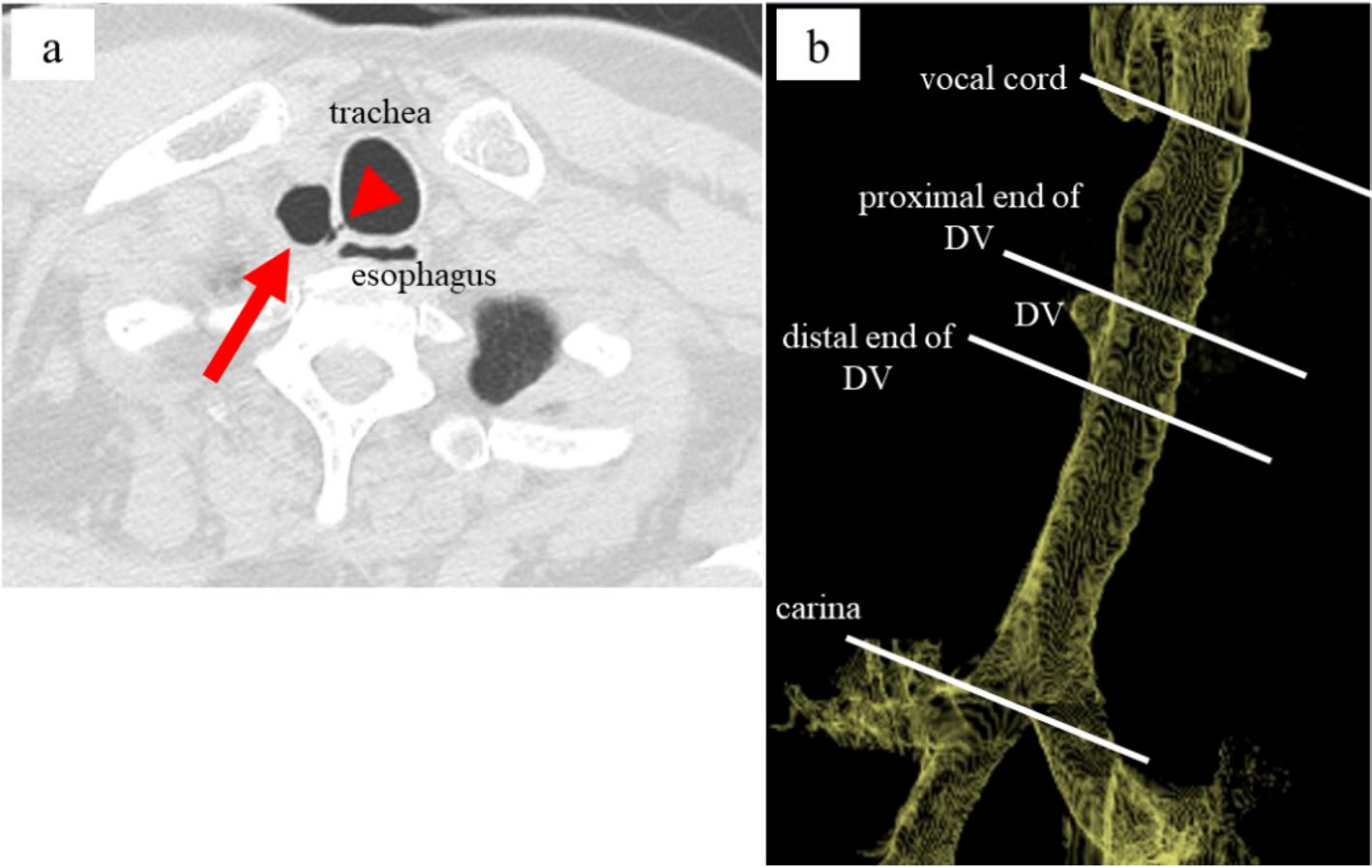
An example of CT image of the tracheal diverticulum and trachea in this study. a Axial view of chest CT image of the tracheal diverticulum (arrow) with an evident orifice (arrowhead). b 3D-CT image of tracheal diverticulum, trachea and anatomical sites relevant to this study. DV: tracheal diverticulum
